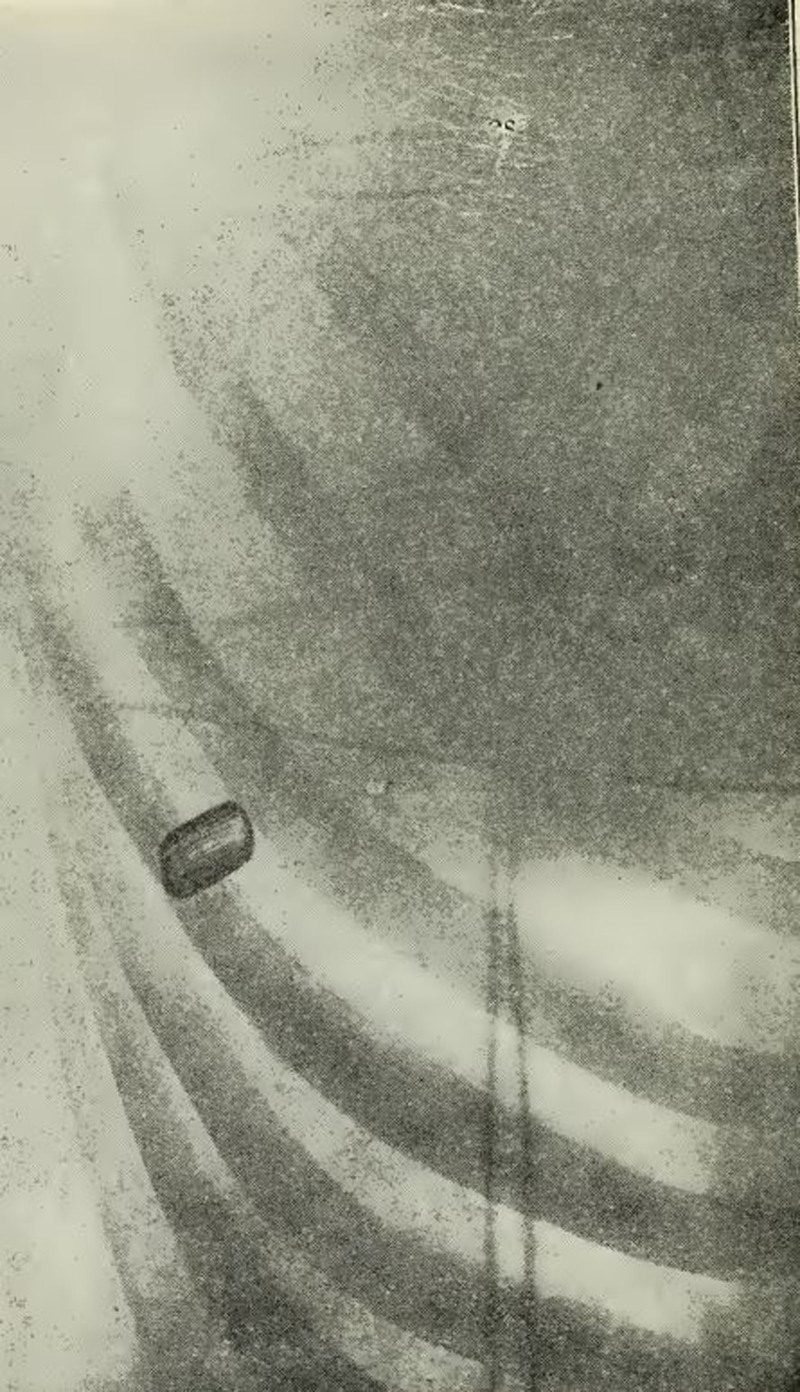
Chest roentgengram of the pistol bullet in Roosevelt’s left chest, taken by Dr. J. S. Jansseen at Johnston Emergency Hospital in Milwaukee.^15^
